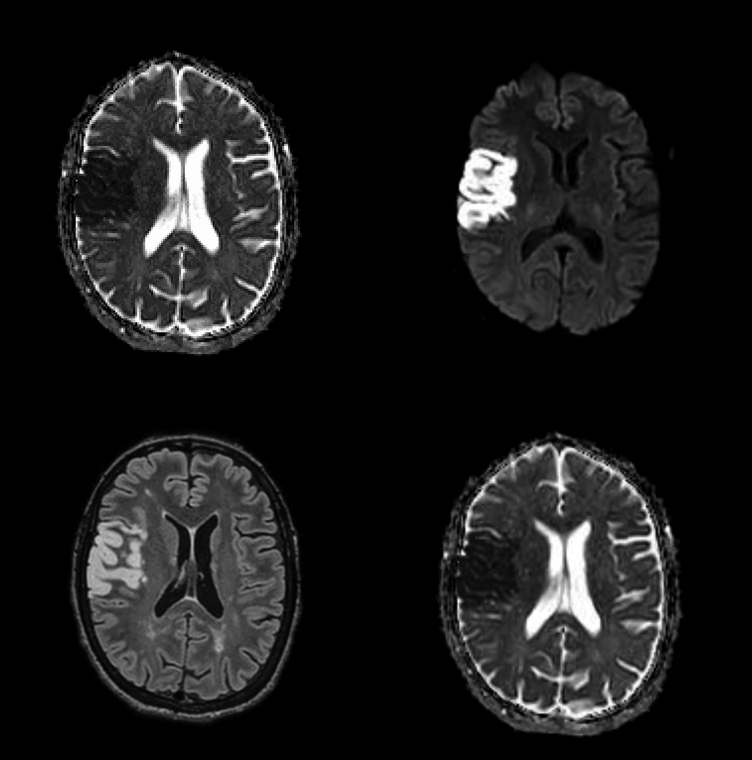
MRI showing a brain infarction in the right frontoparietal region.
